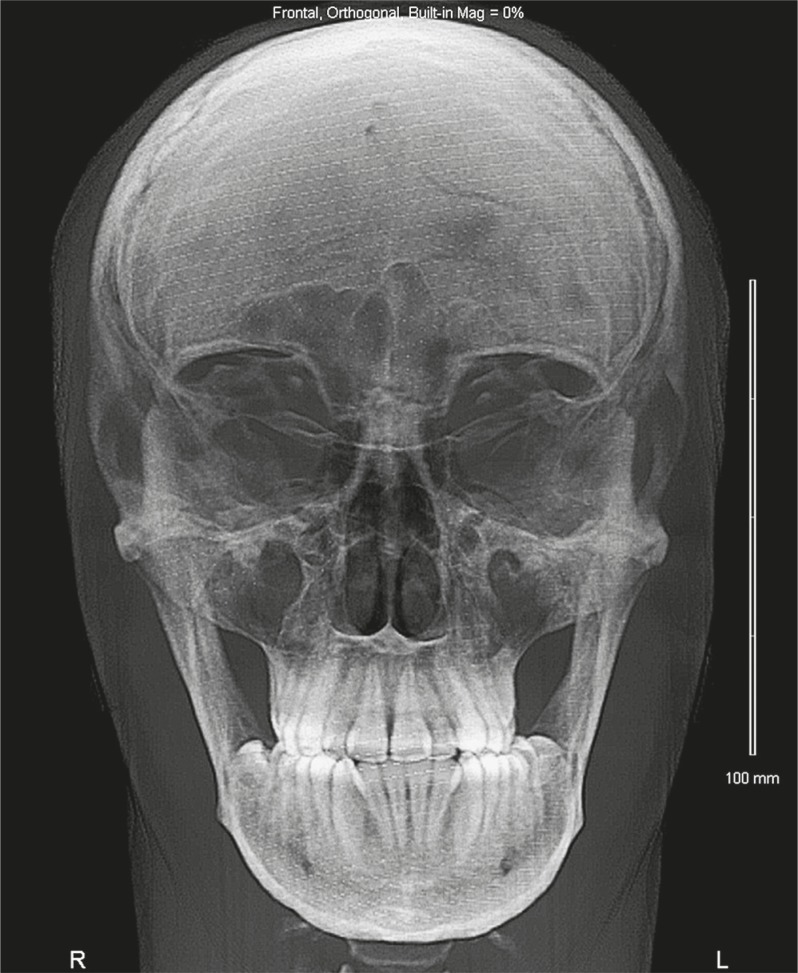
Initial frontal cephalometric radiograph revealing mild
